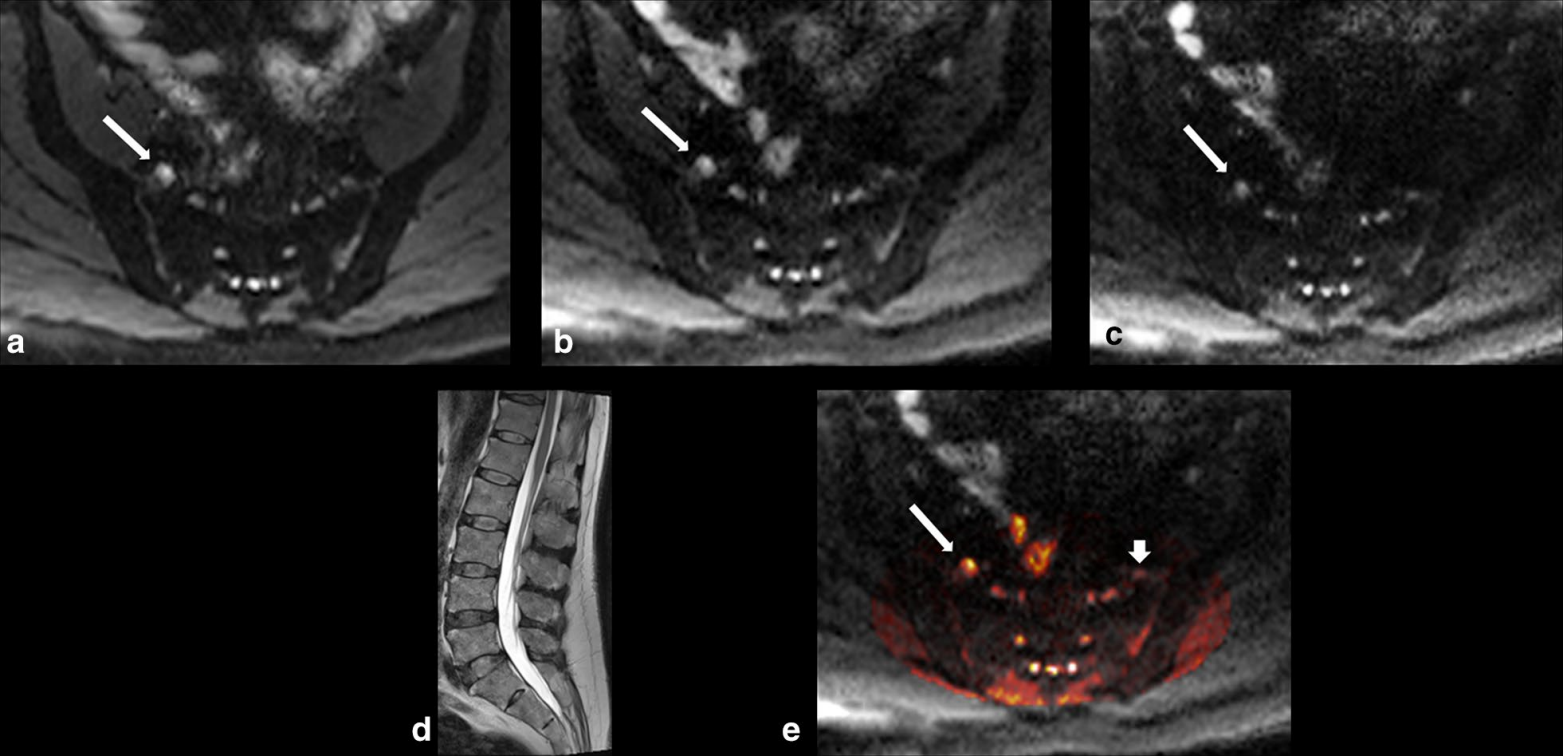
63-year-old man suffering from severe right leg pain and numbness. DWI-RESOLVE images a
